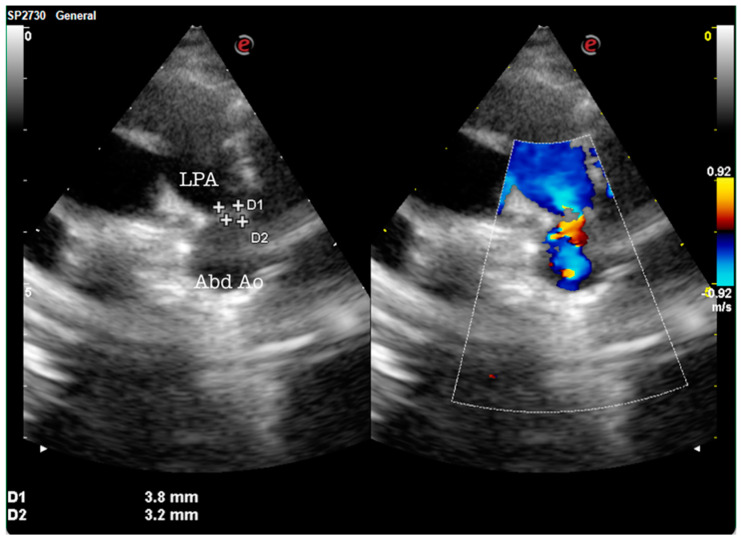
Patent ductus arteriosus (PDA): modified parasternal view showing a moderate tubular/conic patent ductus arteriosus (red flow). LPA—left pulmonary artery; Abd Ao—abdominal aorta.
